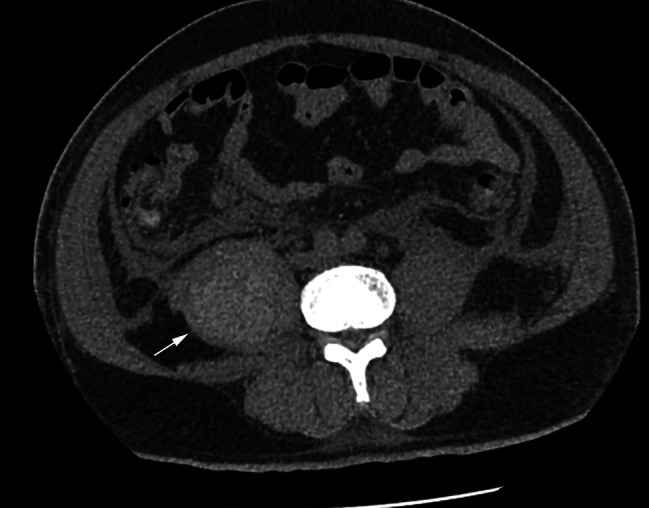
Representative computed tomography (CT) images of the intramuscular hematomas in the right psoas major muscle The CT image showed a lamellar slightly dense shadow of the right psoas major muscle mass (arrow)
